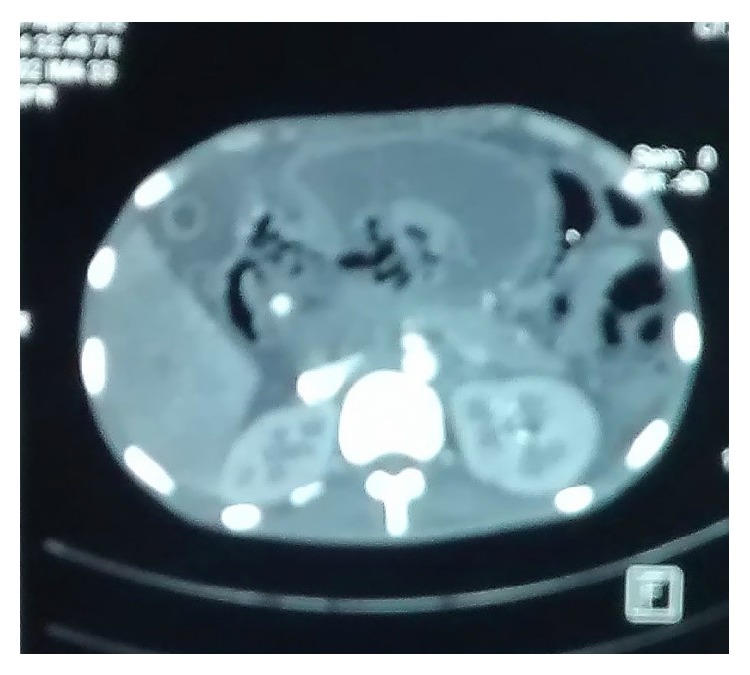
CT abdominal angiography showing gastroduodenal artery aneurysm abutting the wall of the pseudocyst of pancreas.
